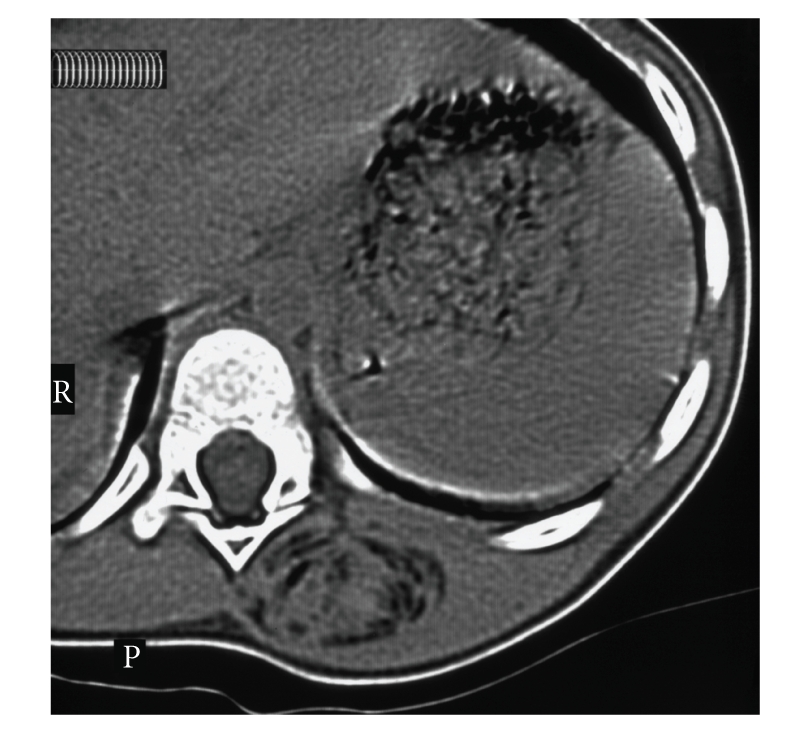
Axial CT image demonstrating a paraspinal mass composed of a mixture of fat and muscle. No bony involvement noted.
